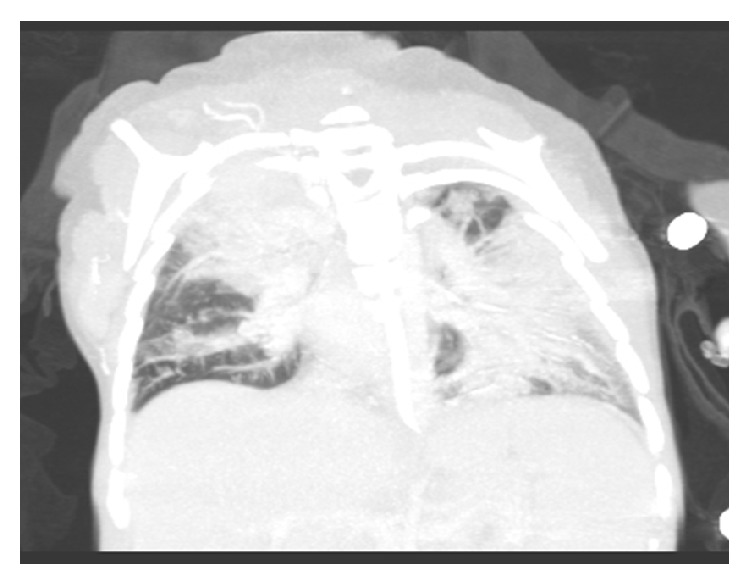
CT scan showing mediastinal enlargement and bilateral infiltrates.
